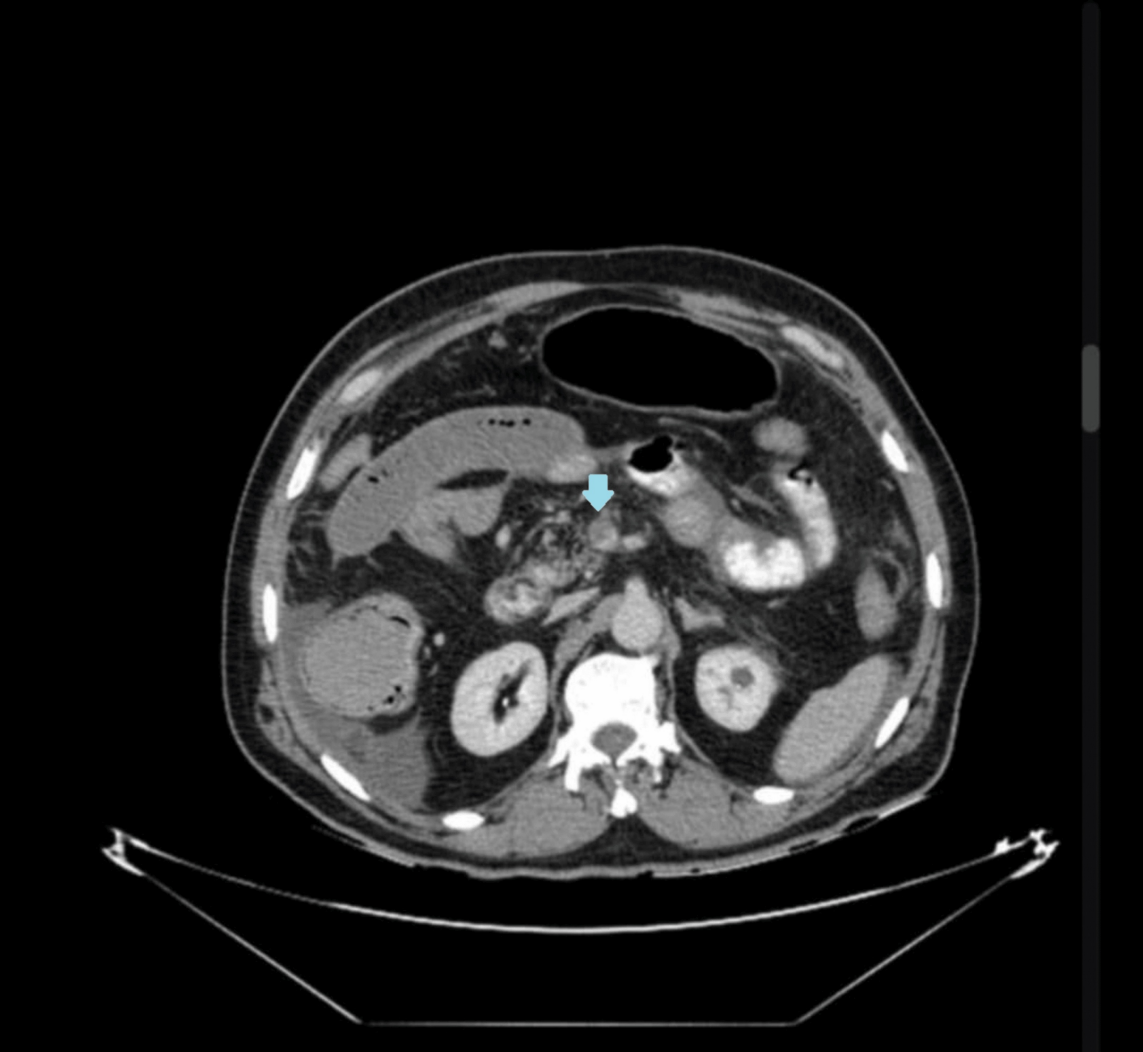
Contrast-enhanced abdominal CT (transverse view) Thrombosis of the superior mesenteric vein with adjacent bowel wall thickening; blue arrow indicates the thrombosed vein.
